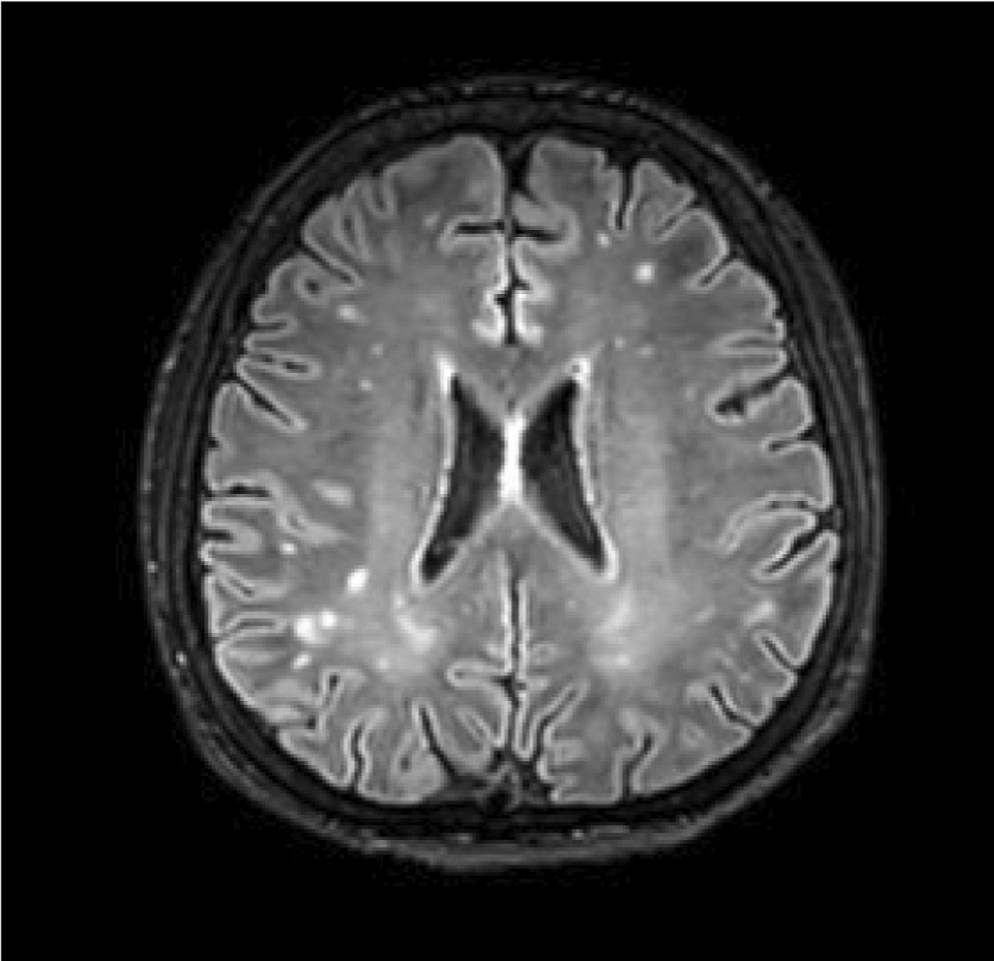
FIGURE 1 Patient 1. Parenchymal lesions in both cerebral hemispheres, asymmetric, FLAIR hyperintense with an inflammatory appearance.
